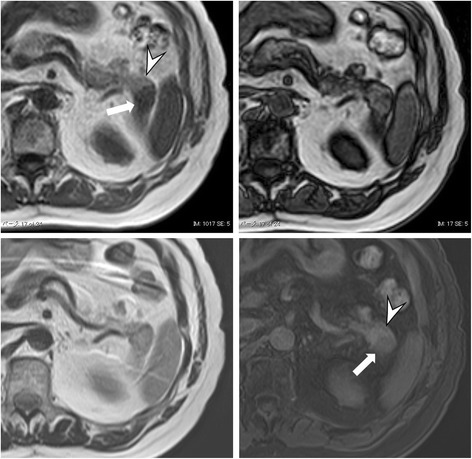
In-phase T1-weighted image (*upper left*) clearly shows the boundary between the pancreatic tail (*arrowhead*) and the spleen (*arrow*). On out-of-phase T1-weighted image (*upper right*), a fat plane between the pancreatic tail and splenic tissue could not be identified and thus suggested fusion. T2-weighted image could not identify the fused border. Fat-suppressed T1-weighted image after gadolinium-ethoxybenzyl-diethylenetriamine pentaacetic acid (*lower right*) differentiated between the two tissues based on their contrast differences
